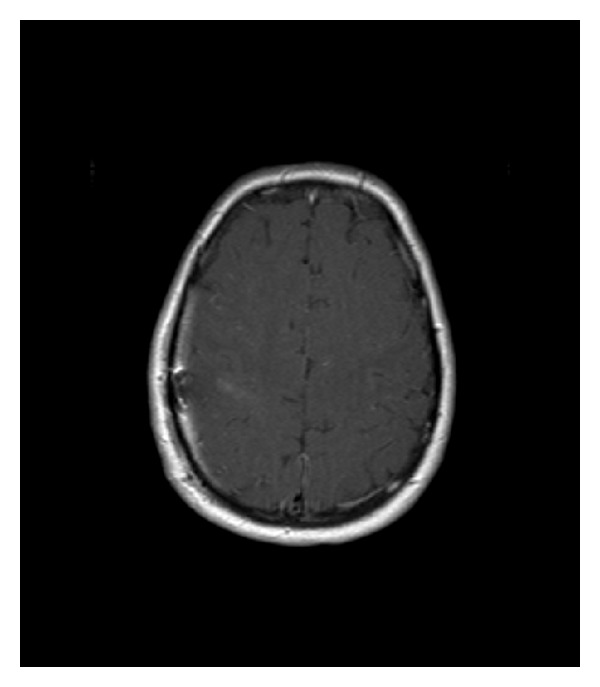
Postoperative MRI showing that the tumor has been totally removed.
